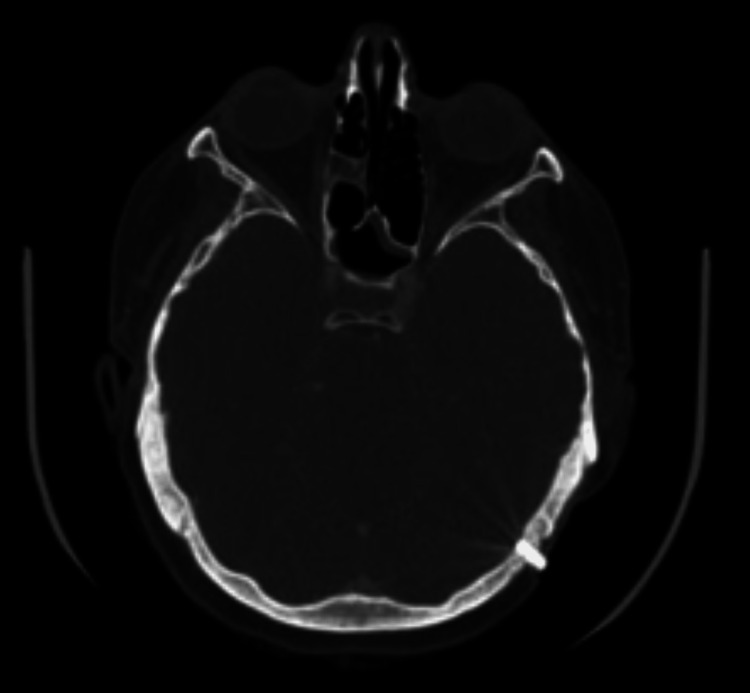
Left BAHA implant visualized on CT scan prior to bisphosphonate therapy on June 23, 2010 BAHA: Bone-anchored hearing aids.
